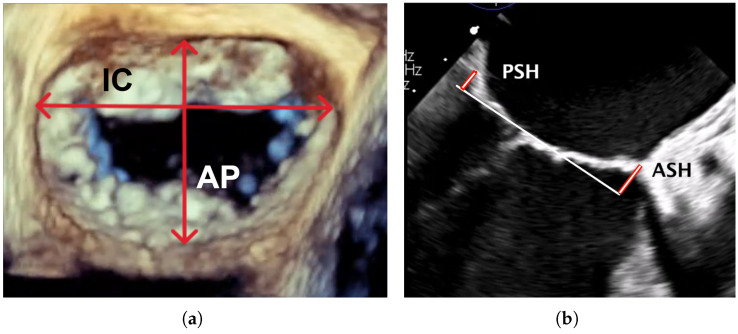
Parameters used to construct the geometry of the mitral valve annulus. (a) Anteroposterior (AP) and intercommissural (IC) distance measurements of the annulus from a surgeon’s view ultrasound image. (b) Anterior saddle height (ASH) and posterior saddle height (PSH) measurements (in red) of the annulus from an ultrasound image. The white line indicates the valvular plane.
